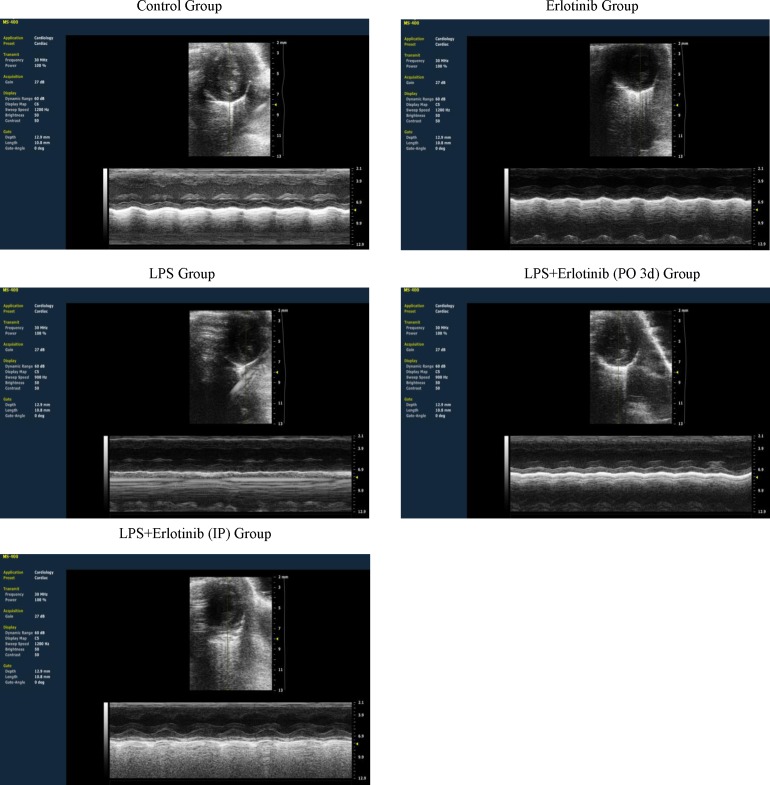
The representative left ventricle section view of cardiac ultrasound in each group
